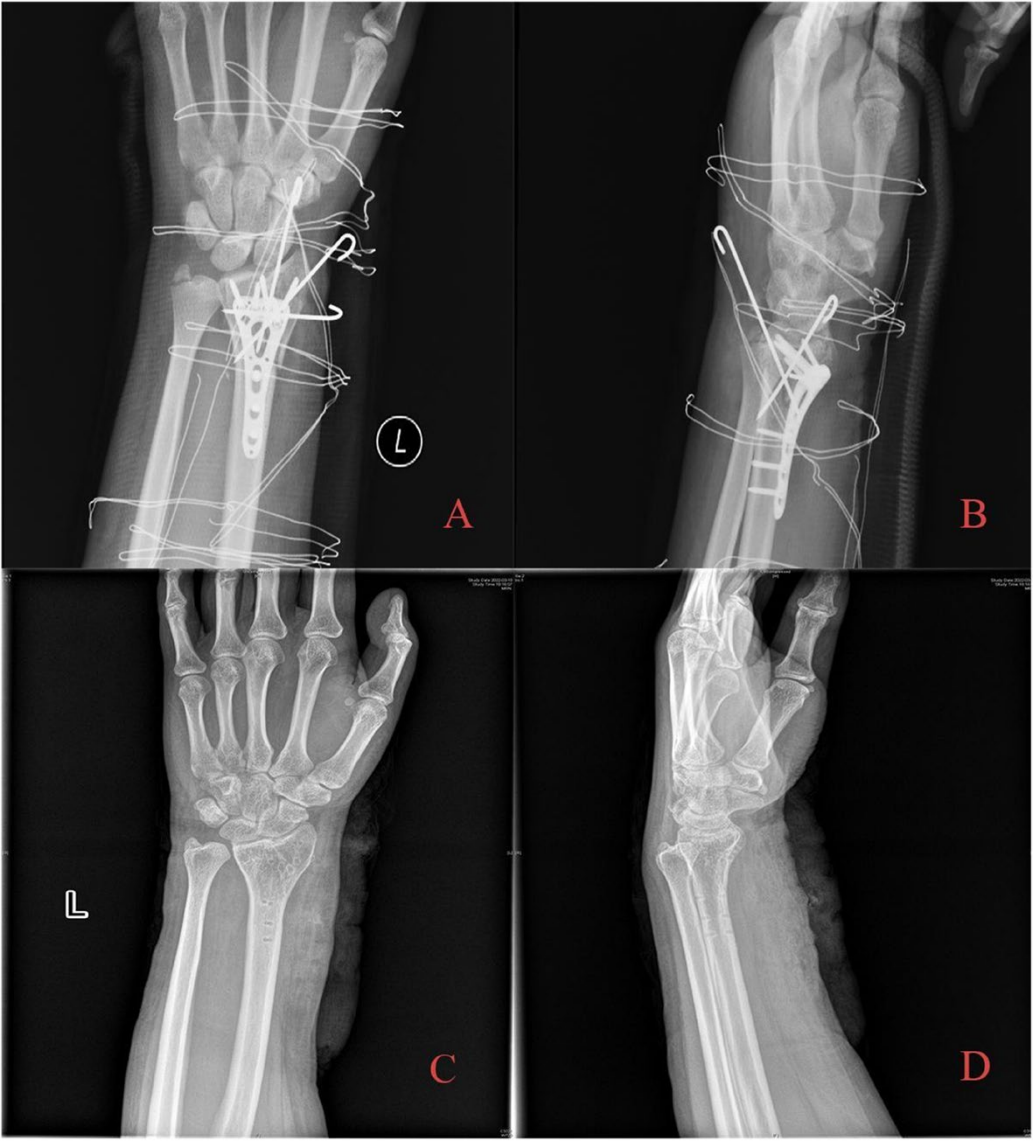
During the operation, it was found that there was a bone defect at the fracture end after indirect reduction. Allogeneic bone grafting was performed, Kirschner wire was used to fix the free bone fragment, and VLP was used to fix the fracture. A Immediate postoperative AP radiography; B Immediate postoperative lateral radiography; C AP radiography after 18 months of follow-up. Radial height was well maintained, and the articular surface was congruent in general; no obvious articular step-off developed. D Lateral radiography after removal of internal fixation
